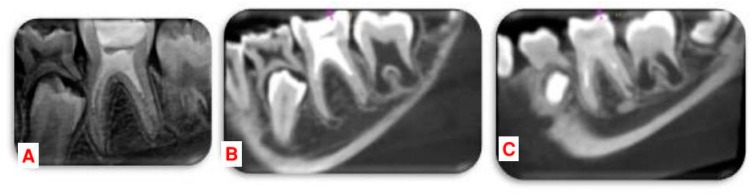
Radiographs at two years (A) Recall 2D radiograph at two years. (B,C) cone-beam computed tomography (CBCT) images in oblique view of the mesial and distal roots.
